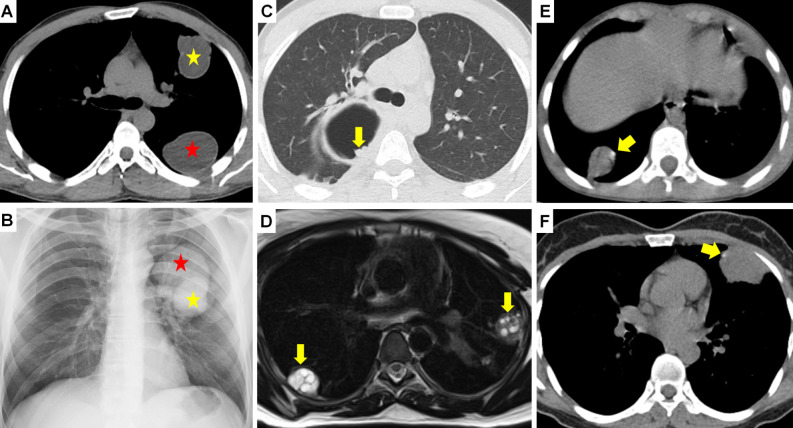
Appearance of 2 simultaneous hydatid cysts (HCs) in the same lung on computed tomography (CT) (A), and the “cyst-in-cyst or silhouette sign” appearance where they overlap and create a silhouette effect on chest radiography (B) (yellow asterisk indicates the anterior cyst, red asterisk indicates the posterior HC). Axial CT (C) shows a ruptured HC and daughter vesicles within the cavity (arrow). T2-weighted MRI (D) shows a multivesicular hyperintense lesion appearance formed by daughter vesicles within HCs in the right and left lungs (arrows). Axial CT shows punctate wall calcifications (arrows) indicating the inactivation or chronic process of the HC in the right (E) and left (F) lungs.
